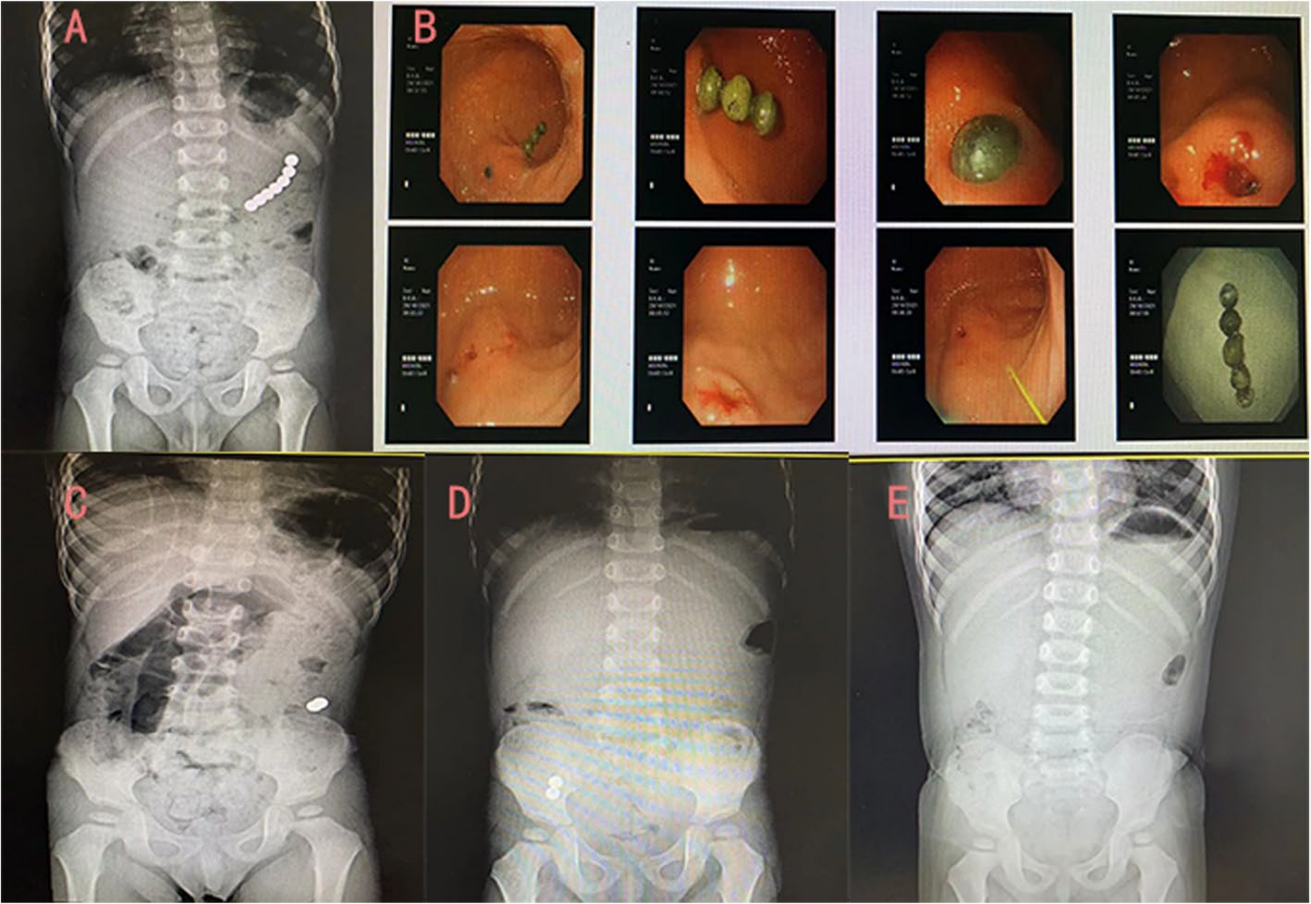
The x-ray showed that the foreign bodies were in the left upper abdomen, and thus, gastroscope was performed. It was found that there were five foreign bodies in the stomach. After being removed by gastroscope, an obvious depression was left on the gastric wall. The patient had no symptoms of pneumoperitoneum and peritonitis, so conservative treatment led to the two remaining foreign bodies being discharged through the anus
